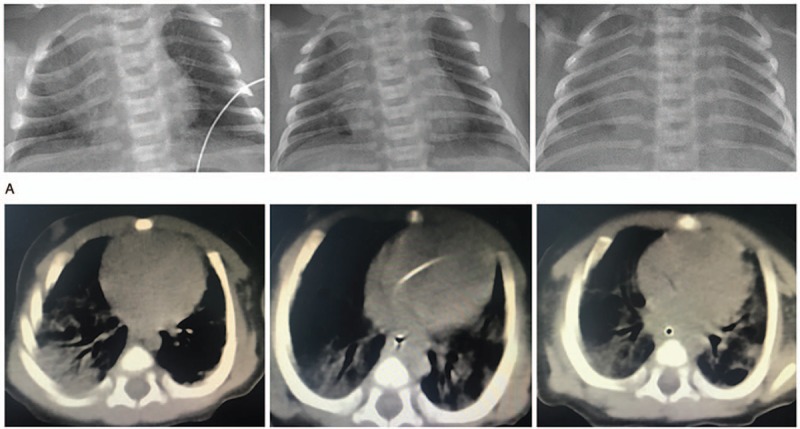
Chest radiographs and CT scan of the patient. (A) Chest radiographs taken at days 9, 16, 28 of life demonstrating a substantial improvement in pneumothorax and continuous deterioration to bilateral diffuse interstitial pneumonia. (B) CT scan taken on day 32 of life. CT = computed tomography.
